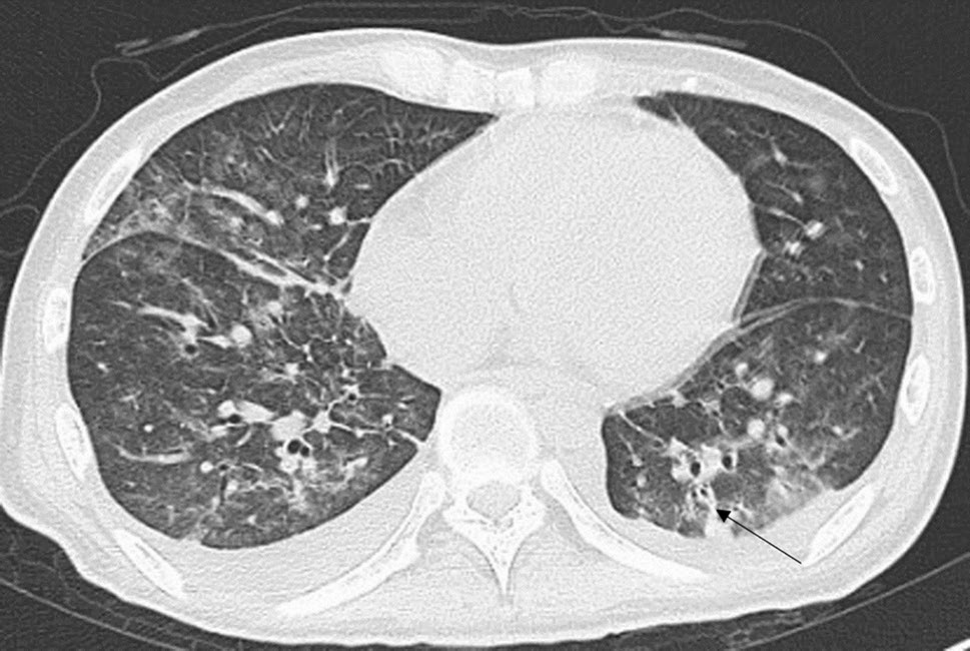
A 29-year-old man with bacterial pneumonia under treatment for acute leukemia. High-resolution computed tomography shows patchy ground-glass attenuation with bronchovascular bundle thickening (arrow)
